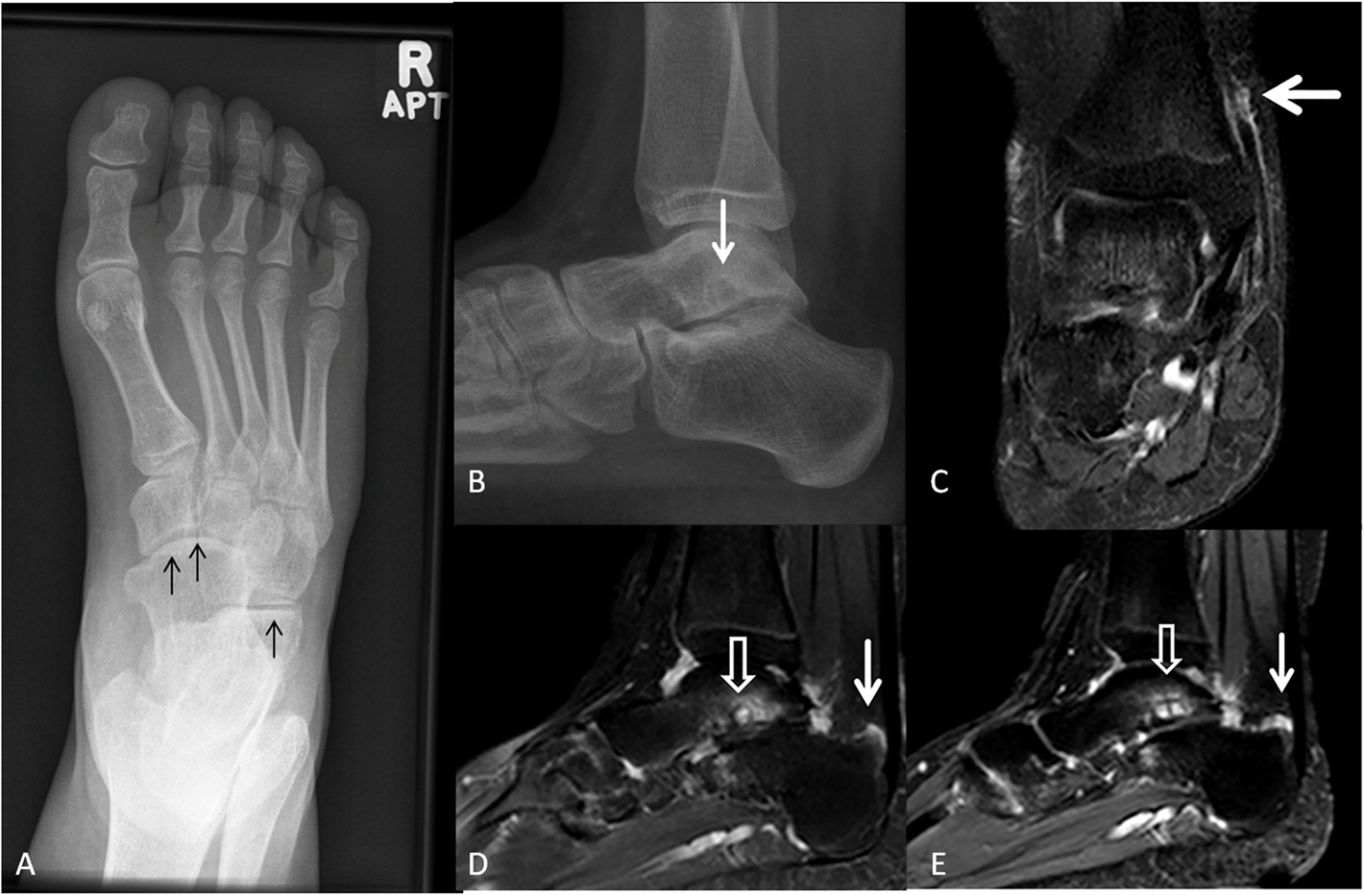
A 14-year-old girl with HA20 presented with pain in her ankles and feet. a Anteroposterior radiograph of the right foot demonstrates joint space narrowing of the talonavicular and calcaneocuboid joints (arrows). Similar but asymmetrical changes are present in the left foot (not shown). b Lateral radiograph of the right ankle demonstrates subtalar joint space narrowing with subchondral sclerosis and subarticular cystic changes of the inferior surface of the talus (arrow). c Coronal STIR of the right ankle demonstrates fluid tracking along the tibialis posterior tendon (arrow) with post gadolinium enhancement (not shown). d Sagittal STIR and e T1-weighted image with fat suppression and intravenous gadolinium administration of the right ankle demonstrate subtalar joint space narrowing and subchondral cystic changes with surrounding edema involving the inferior articular surface of the talus and contrast enhancement (empty white arrow). There is joint space narrowing and small effusion in the tibiotalar, subtalar, and talonavicular joints with synovial contrast enhancement. There is retrocalceneal bursistis (white arrow). Arthritis, tenosynovitis, and retrocalceneal bursistis are likely inflammatory with degenerative changes of the subtalar joint
